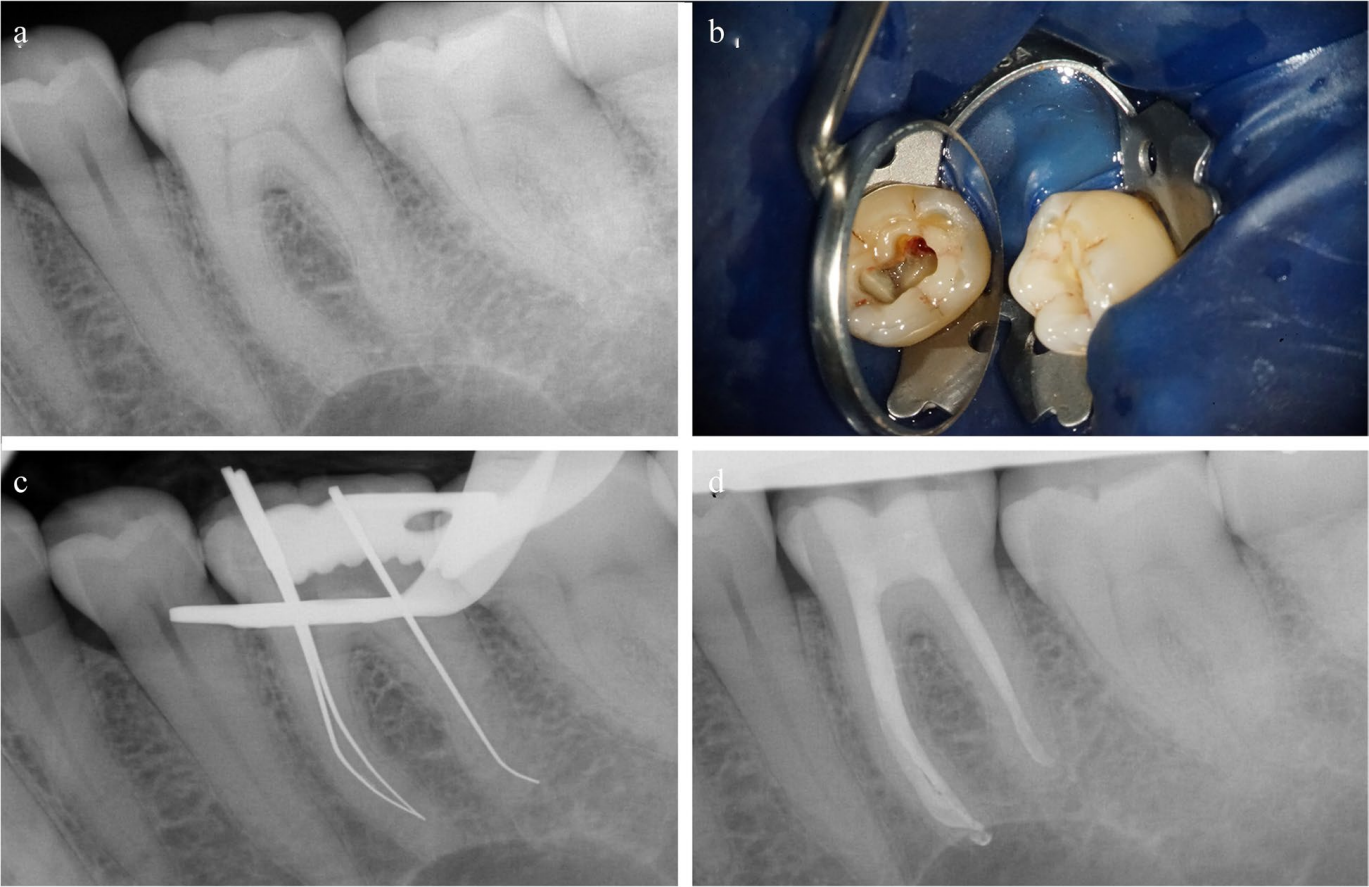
Intra-oral color photograph and periapical radiographs. a IOPAR pre-endodontic treatment. b Access cavity and depiction of tertiary dentine. c IOPAR to control the working length. d IOPAR post-endodontic treatment
